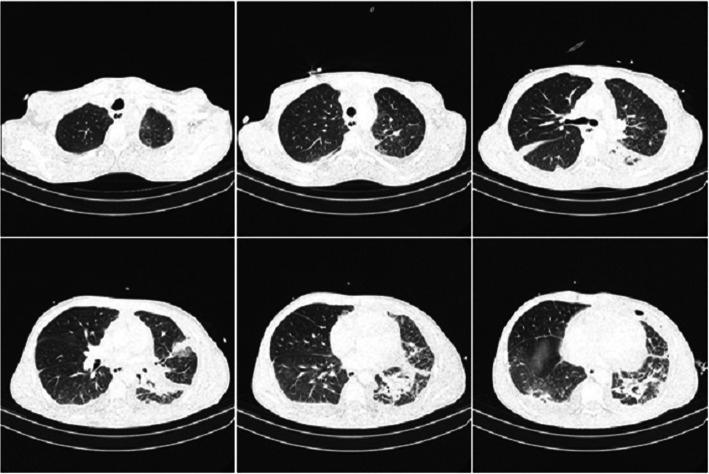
Computed tomographic scan of the chest of the patient after targeted segmental recruitment (36th day of stay in the intensive care unit)
